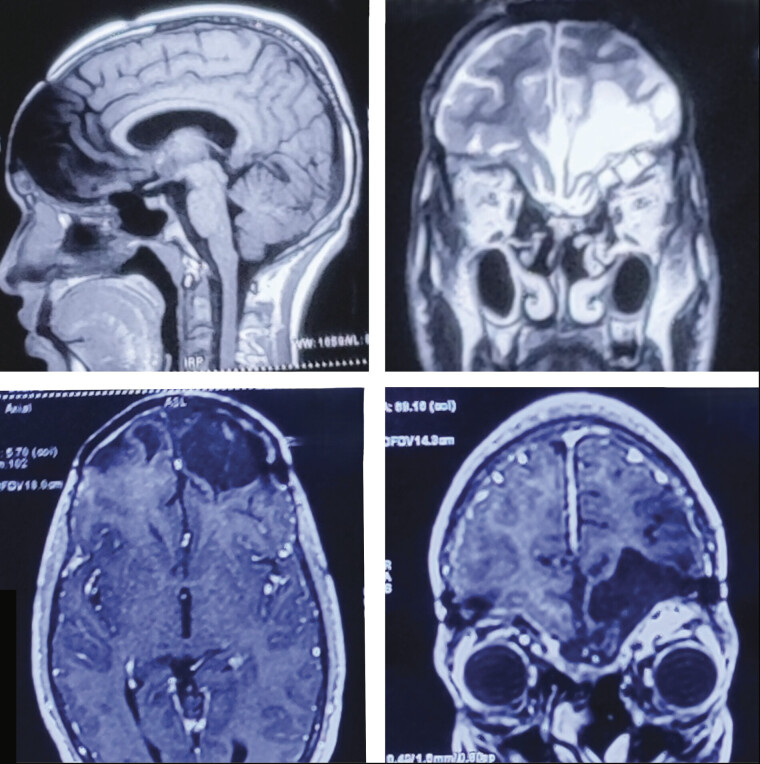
Magnetic resonance imaging (MRI) of brain at 1-year follow-up. Clockwise—T1-weighted sagittal, T2-weighted coronal, T1-weighted post-contrast coronal, and T1-weighted post-contrast axial images showing gliosis/encephalomalacial changes in bilateral basifrontal and left high frontal region with no evidence of any recurrence or any contrast enhancement.
